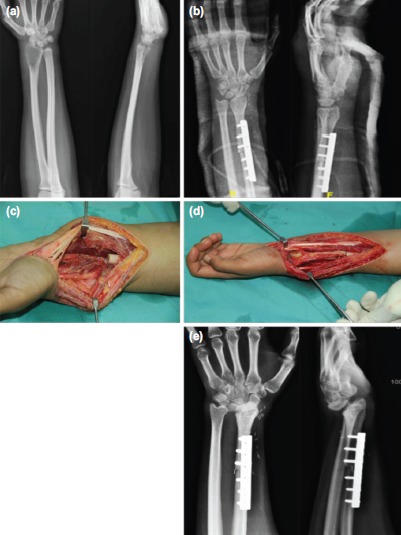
Mobile wrist reconstruction in a 30 year-old lady teacher with left distal radius giant cell tumour. (a) Pre-operative radiograph. (b) Early post-operative radiograph showing good reconstruction. (c) Intra-operative photograph showing bony defect post-wide resection of left distal radius tumour. (d) Wrist reconstruction with non-vascularised proximal fibular graft. (e) Left wrist subluxation noted three years after surgery with arthritic changes, but daily activities were unaffected.
